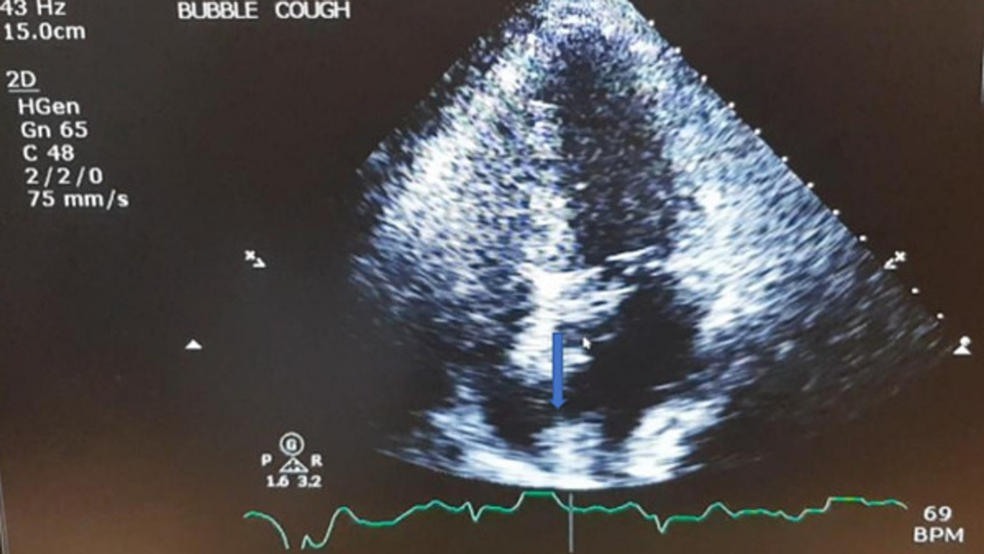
Bubble study echocardiogram showing a PFO. PFO: patent foramen ovale
